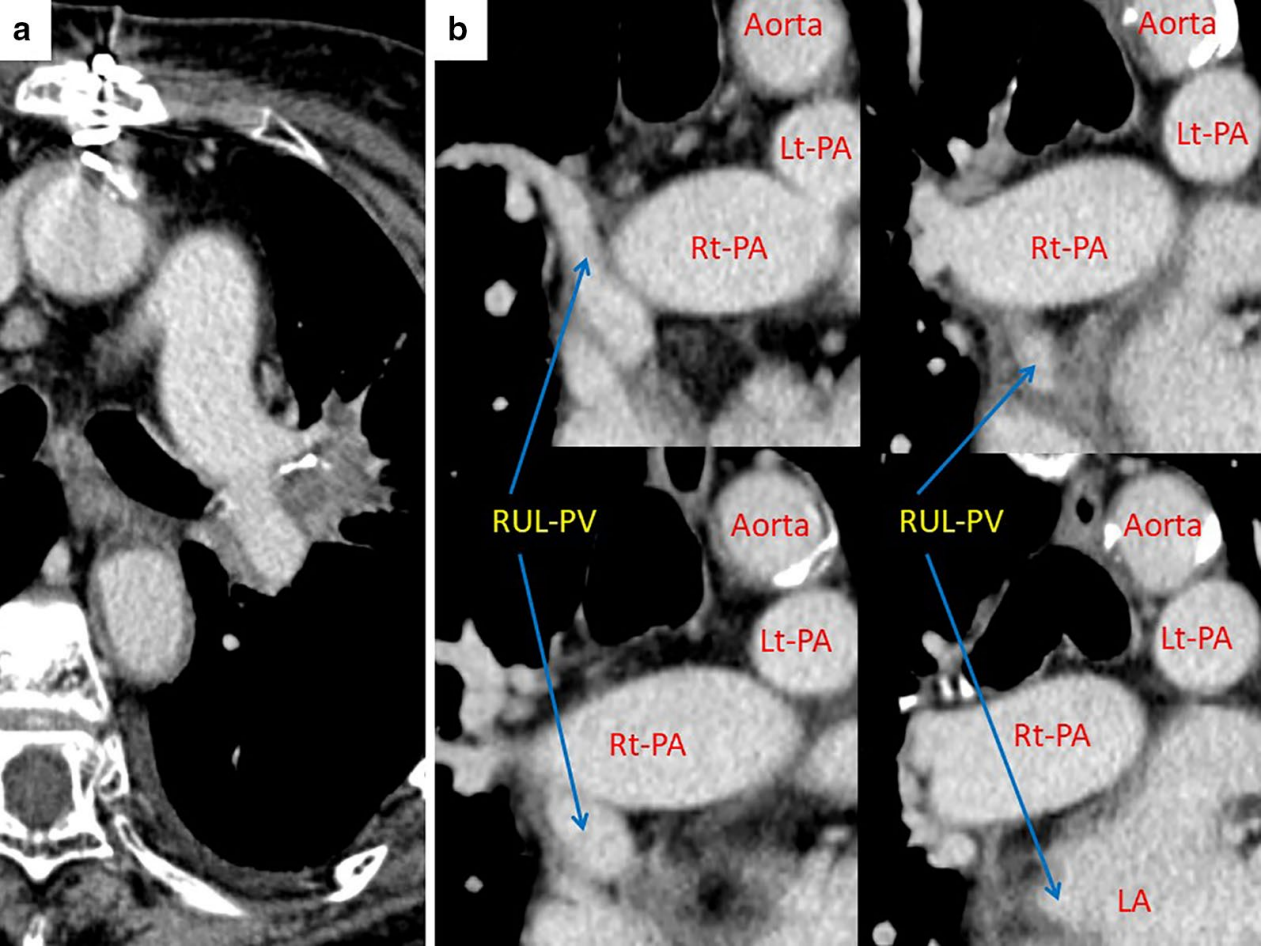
Enhanced chest computed tomography after induction chemoradiation therapy (a) revealed a 3.2-cm solid mass in the left-upper lobe that showed a partial response according to the response evaluation criteria in solid tumor (− 49% in size). The enhanced chest computed tomography (coronal view) after surgical correction of the partial anomalous pulmonary venous connection in right-upper lobe (b) showed the right-upper lobe pulmonary vein draining into the left atrium. *RUL* right-upper lobe, *RML* right-middle lobe, *PV* pulmonary vein, *PA* pulmonary artery, *Rt* right, *Lt* left
